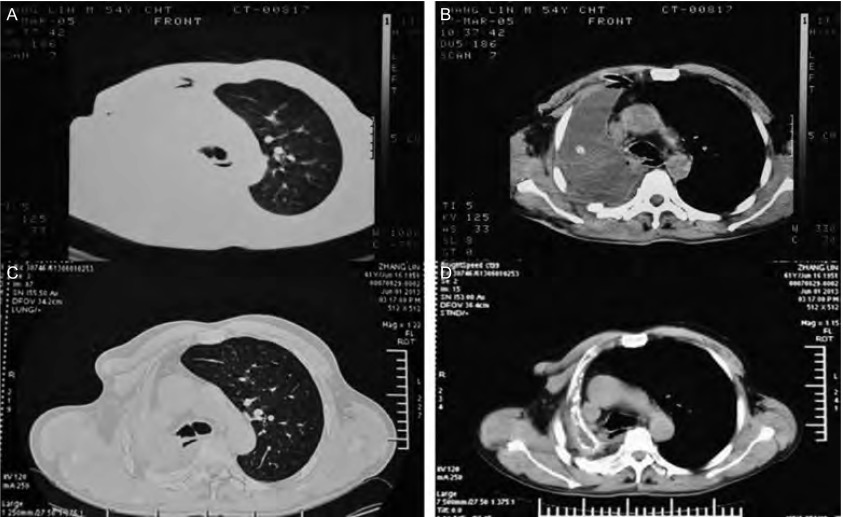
术后CT。A、B：右全肺切除术后，右侧胸腔积液、见引流管截面影（2005年3月）; C、D：纵隔右移，右侧胸廓塌陷，右全肺切除术后所见，未见肿瘤复发、转移征像（2013年5月）。 Postoperative CT scan of the chest. A, B (March 2005): After right pneumonectomy, the pleural effusion is on the right side, shadow of the chest drainage tube section is seen; C, D (May 2013): After right pneumonectomy, the mediastinum has been moved to the right with right chest collapsed. There is no expression of the tumor relapse or metastasize.
